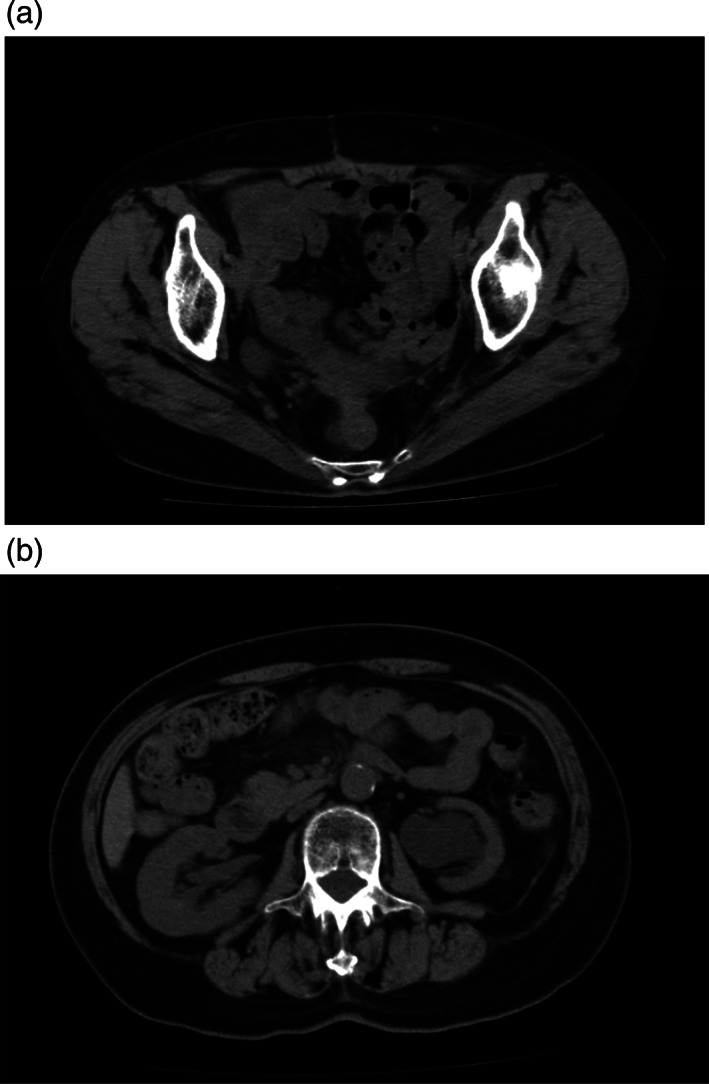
Four months after the second drainage, neither the lymphocele nor right hydronephrosis were detected by computed tomography (a, b)
